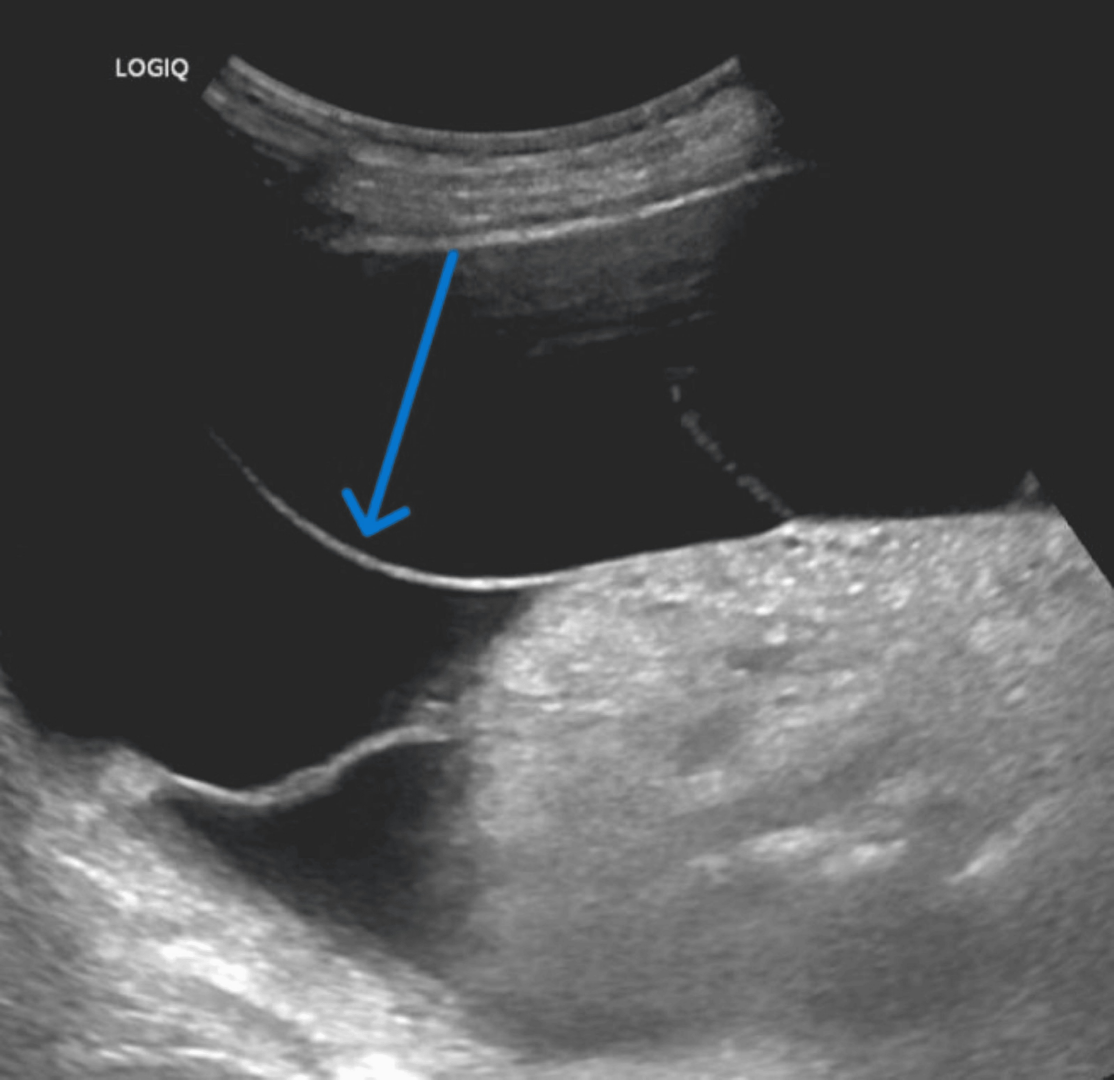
Right upper quadrant ultrasound prior to drain placement and tPA dwells. Ultrasound demonstrates a few of the patient's numerous loculations in this region with a large fluid collection. tPA: alteplase
